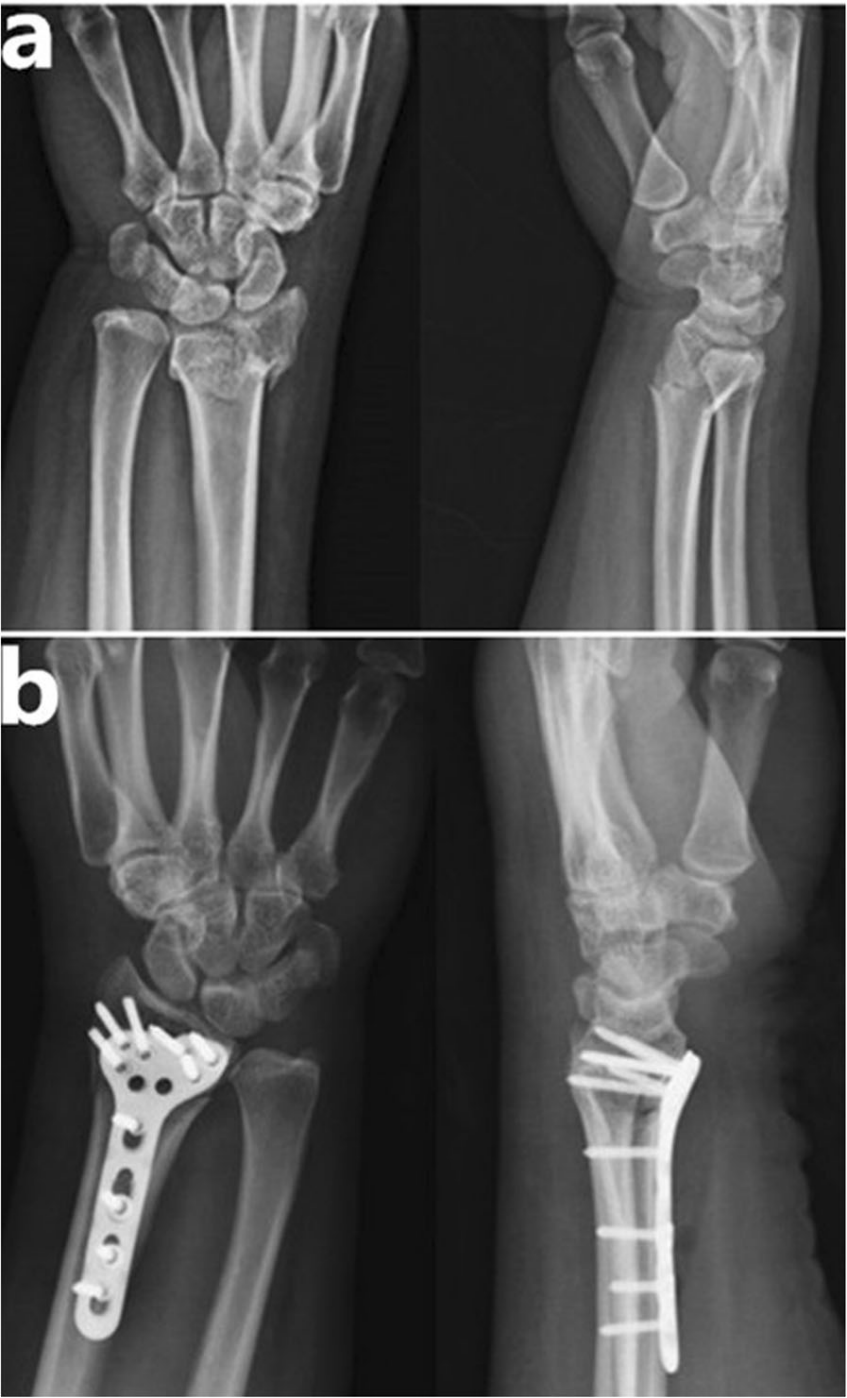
a Anteroposterior and lateral radiographs of a 34-year-old female with AO C1 fracture before operation. b Posteroanterior and lateral radiographs taken immediately after operation showed that anatomic reduction had been obtained using a VLP
